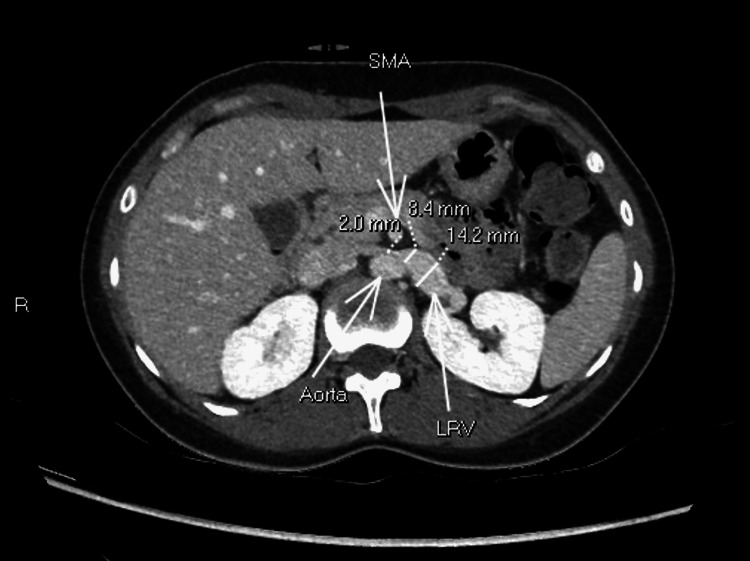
Axial CT images reveal a markedly narrowed LRV (> 50 %) between the aorta and SMA. Pre-stenotic dilatation of the left renal vein is also seen. LRV: Left renal vein, SMA: Superior mesenteric artery
